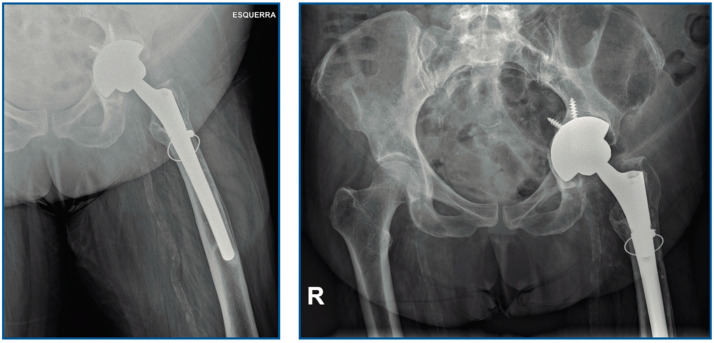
Hip radiography showing the implanted prosthesis.
